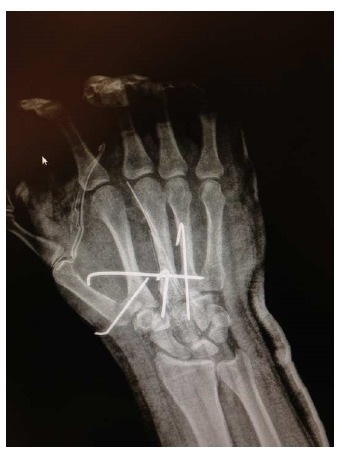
Post-operative radiographs showing the reduced second, third, and fourth carpometacarpal joints.
